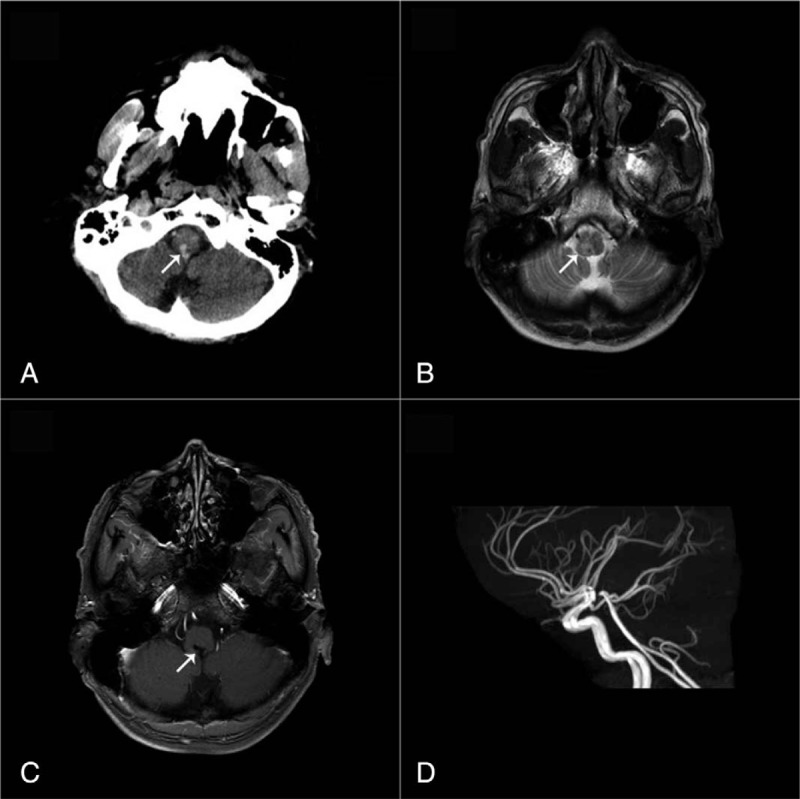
Brain images. Brain CT demonstrated a hemorrhagic lesion in dorsal and medial medulla oblongata with hemorrhage rupturing into fourth ventricle (A; brain MRI with T2 sequences demonstrated hyperintensity in medulla oblongata (B); at 6-month follow-up MRI showed a malacic lesion (C); non-contrast enhanced MRI demonstrated no abnormal areas of enhancement, and negative findings were seen on follow-up MRA (D). CT = computed tomography, MRA = magnetic resonance angiography, MRI = magnetic resonance imaging.
